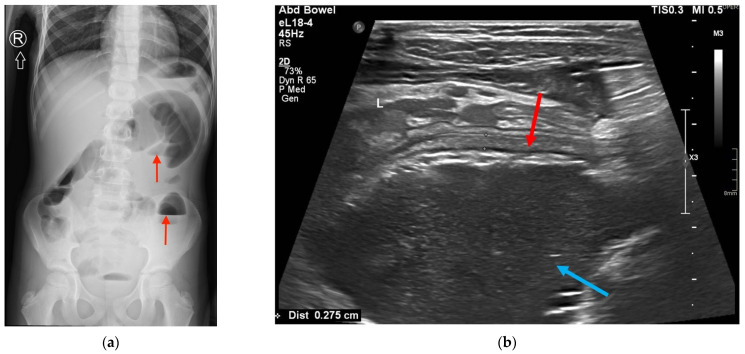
(a) Single air-fluid levels on X-ray (red arrows); (b) US examination showing intestinal wall edema (red arrow) and distended, fluid-filled lumen of the intestine (blue arrow).
